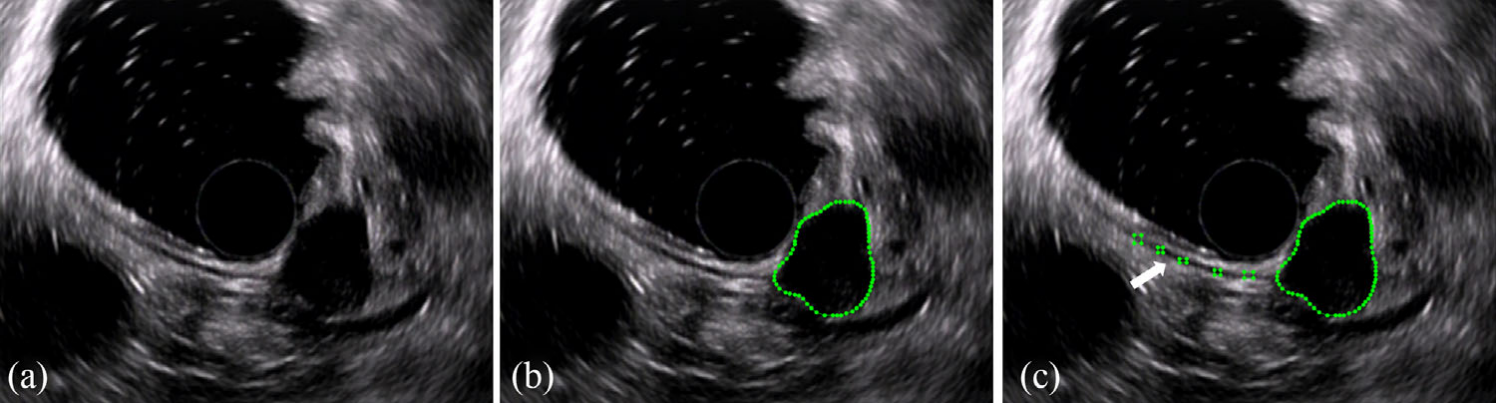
ROI segmentation for radiomic analysis: (a) original EUS image; (b) segmentation of tumor region; and (c) segmentation of tumor region and muscularis propria region (arrow). EUS, endoscopic ultrasonography; ROI, region of interest.
